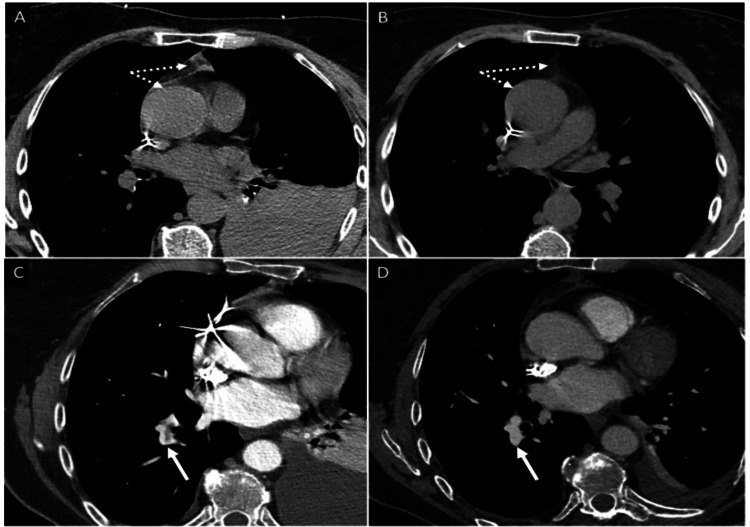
CT scan findings of aortic IMH and PE In panel A, the dotted arrows indicate the location of aortic IMH during the acute phase, characterized by high attenuation and thickening of the aortic wall (about 3-3.5 mm) and associated with fat stranding of the periaortic anterior fat. Panel B shows the IMH (dotted lines) at one month. The aortic wall is normal, and the fat attenuation is almost normalized. In panel C, the white arrow indicates the location of PE. Panel D shows the PE (white arrow) at one month, demonstrating complete resolution. IMH: intramural hematoma; PE: pulmonary embolism
